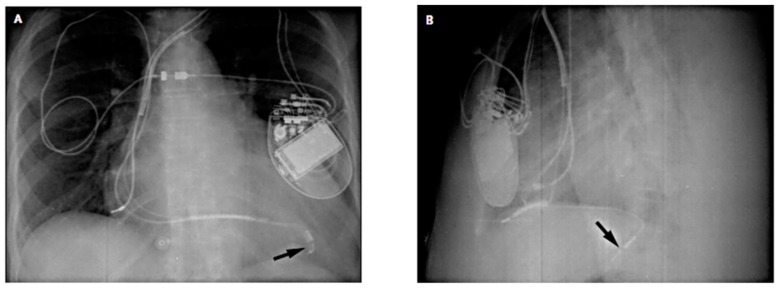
Chest radiographs showing lead placements. (A) Posteroanterior and (B) lateral chest radiographs showing tip of a large left ventricular lead (arrows) in a tributary of the middle cardiac vein. Despite proximity of the lead to the left hemidiaphragm, phrenic nerve stimulation did not take place. The right ventricular lead points anteriorly toward the rib cage. Reproduced with permission from reference [29].
